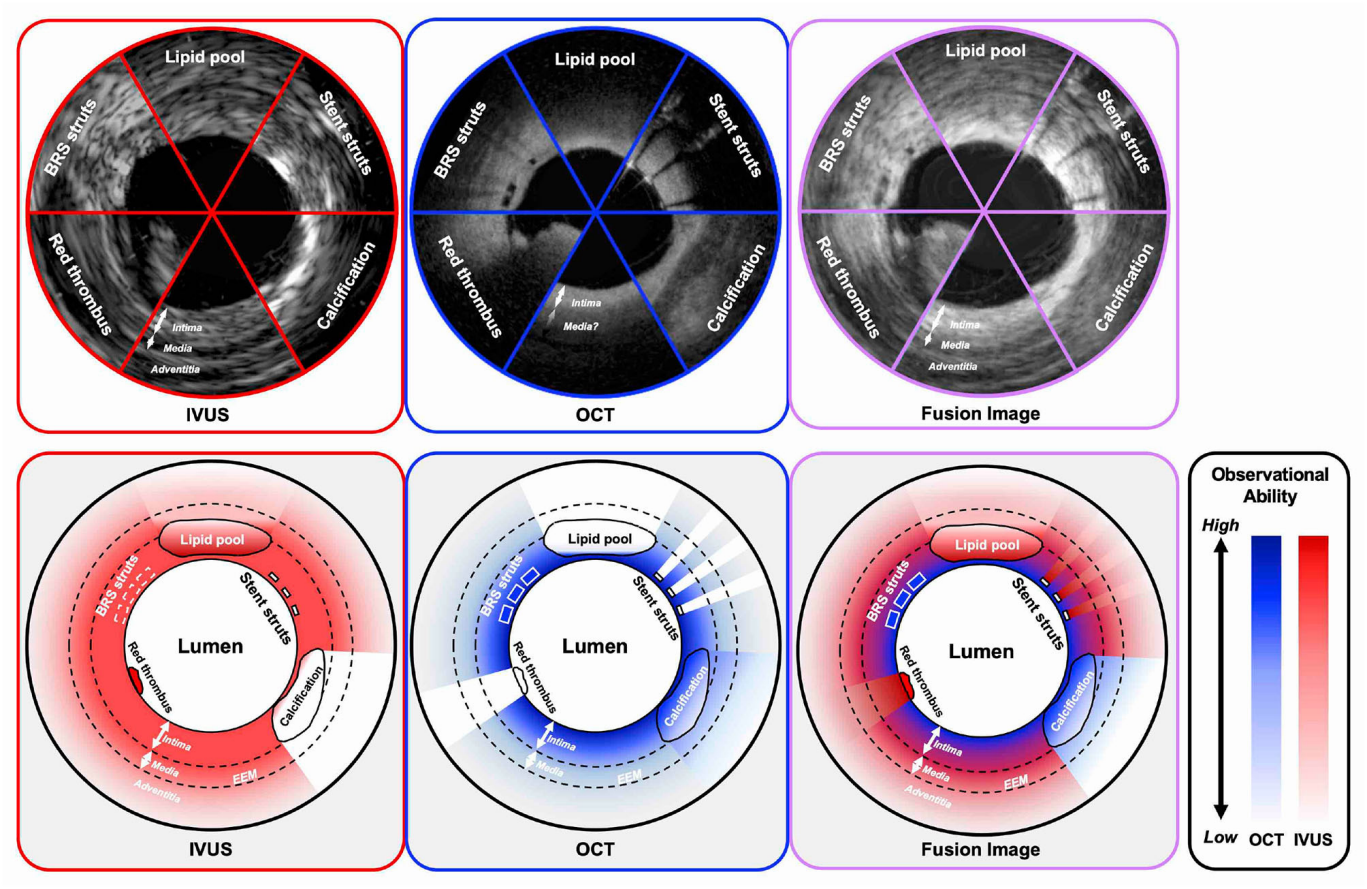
Observational abilities of IVUS and OCT in the settings of several intracoronary structures. IVUS indicates both grayscale IVUS and IVUS with radiofrequency analysis. IVUS, intravascular ultrasound; OCT, optical coherence tomography; BRS, bioresorbable scaffold; EEM, external elastic membrane.
